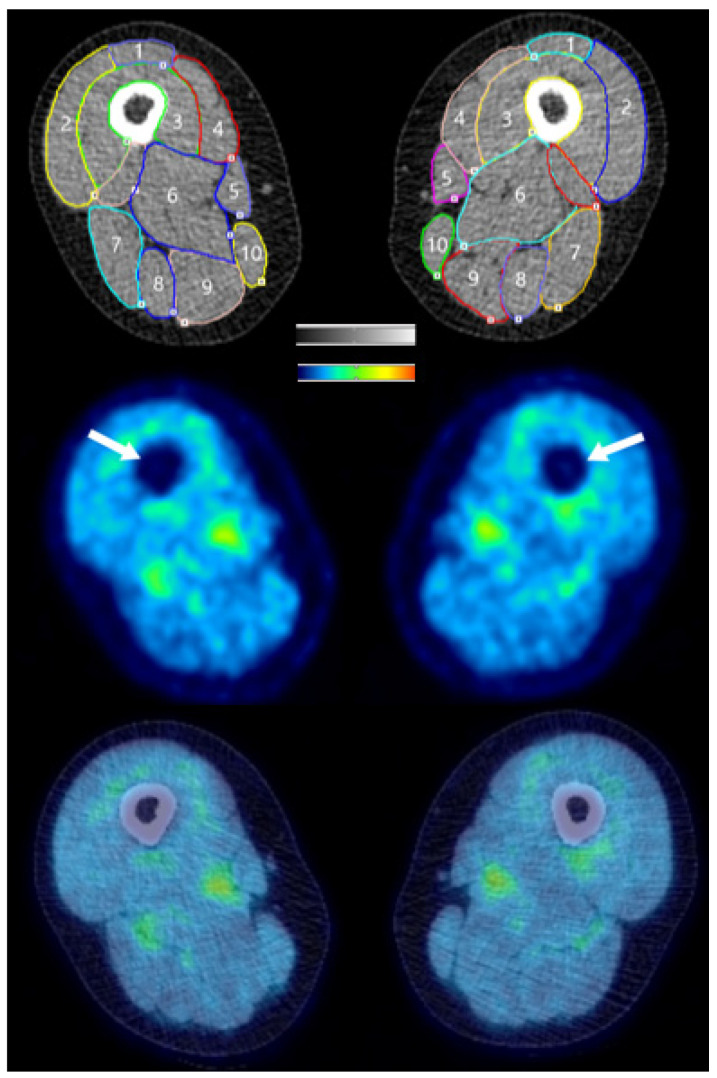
Cross-sectional slice of a CT scan taken at the mid-thigh level in Subject 4. Regions of interest (ROIs) were drawn on the computer tomography (CT) scan (top image) to indicate the different skeletal muscles of the lower limb. 1 = Rectus Femoris, 2 = Vastus Lateralis, 3 = Vastus Intermedius, 4 = Vastus Medialis, 5 = Sartorius, 6 = Adductor Magnus (not included in the analysis), 7 = Biceps Femoris, 8 = Semitendinosus, 9 = Semimembranosus, 10 = Gracilis. The ROIs were copied to the corresponding positron emission tomography (PET) image (middle image). The white arrows indicate the femur in the middle image. The bottom image is an example of a PET scan co-registered to a CT scan. Red denotes the greatest signal intensity, followed by yellow, green, and blue.
